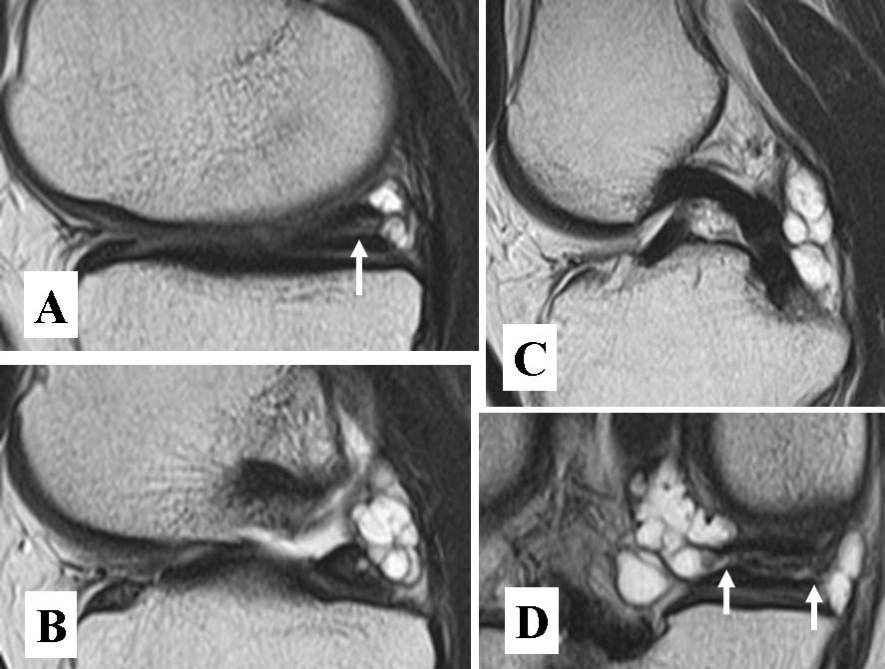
Sagittal images (A,B,C) and coronal image (D) of T2 weighted MRI. A horizontal meniscal tear is identified inside the meniscus in Figure 1-A (a white arrow). A multilobulated cyst is located just behind the medial meniscus and the PCL (B, C). The cyst is in communication with the meniscal tear on Figure 1-D (white arrows). Note that the horizontal meniscal tear has not extended to the tibial or femoral surface.
